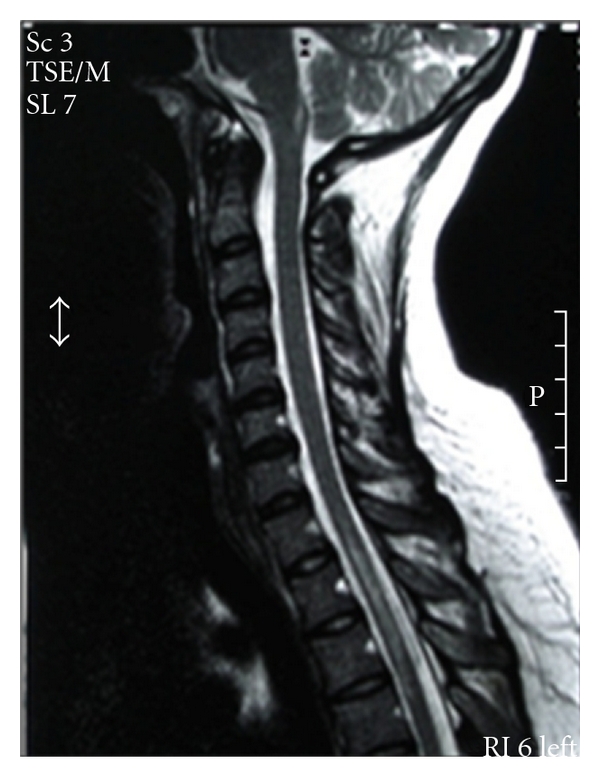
Sagital T2-MRI of the spinal cord showing an extensive enhancing thoracic lesion (Th1-Th3).
